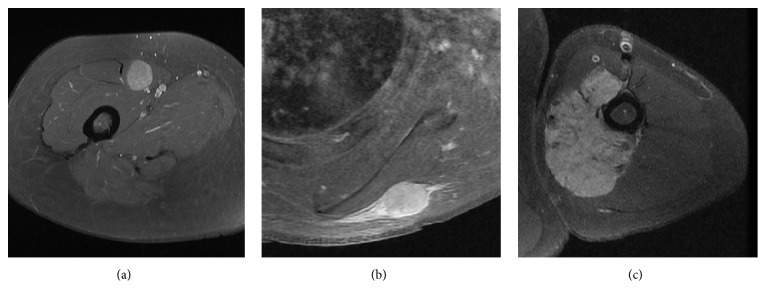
Representative images of different tumor borders on MRI. (a) T1 enhanced MRI of an intramuscular synovial sarcoma with pushing border on MRI. (b) T1 enhanced MRI of an intramuscular undifferentiated pleomorphic sarcoma with infiltrative border on MRI with the tail sign. (c) T1 enhanced MRI of a leiomyosarcoma with infiltrative border on MRI without the tail sign.
